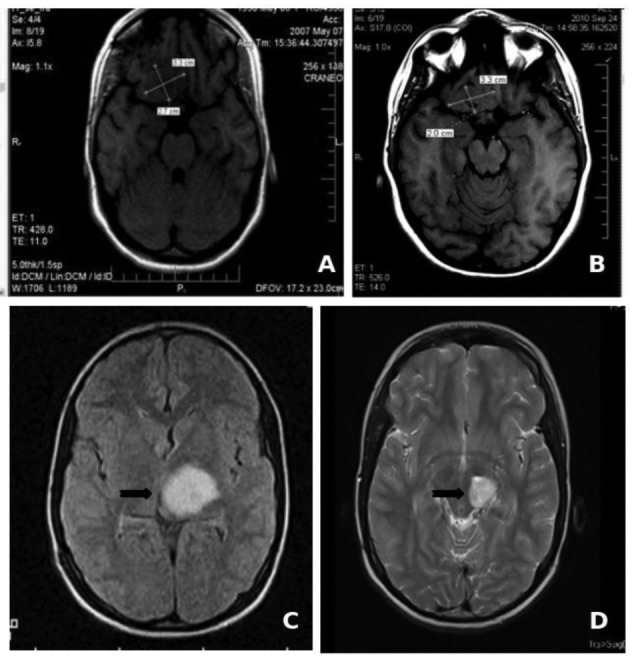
Conventional MRI imaging of Nimotuzumab long-term treated tumors for two pediatric pontine glioma patients, showing a prolongued stable disease (SD) after more than 100 doses of Nimotuzumab. Patient AFR: (A). May 2007, tumor diameters (TD): 3.3 cm × 2.2 cm; (B). September 2010, TD: 3.3 cm × 2.0 scm. Patient GRA: (C). December 2007; (D). April 2010, black arrows point to tumor images.
